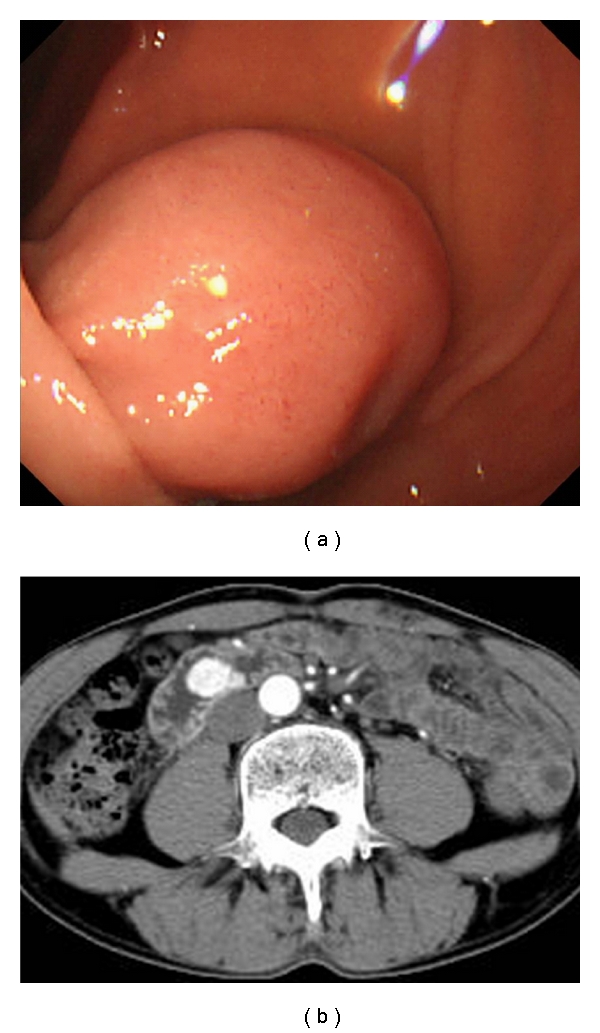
(a) Endoscopic appearance revealed a 20-mm diameter submucosal tumor with a smooth surface in the third portion of the duodenum. (b) Preoperative endoscopic ultrasonography (12 MHz miniature probe). Arrowheads indicate that muscle layer was preserved beneath the tumor without an extramural component.
